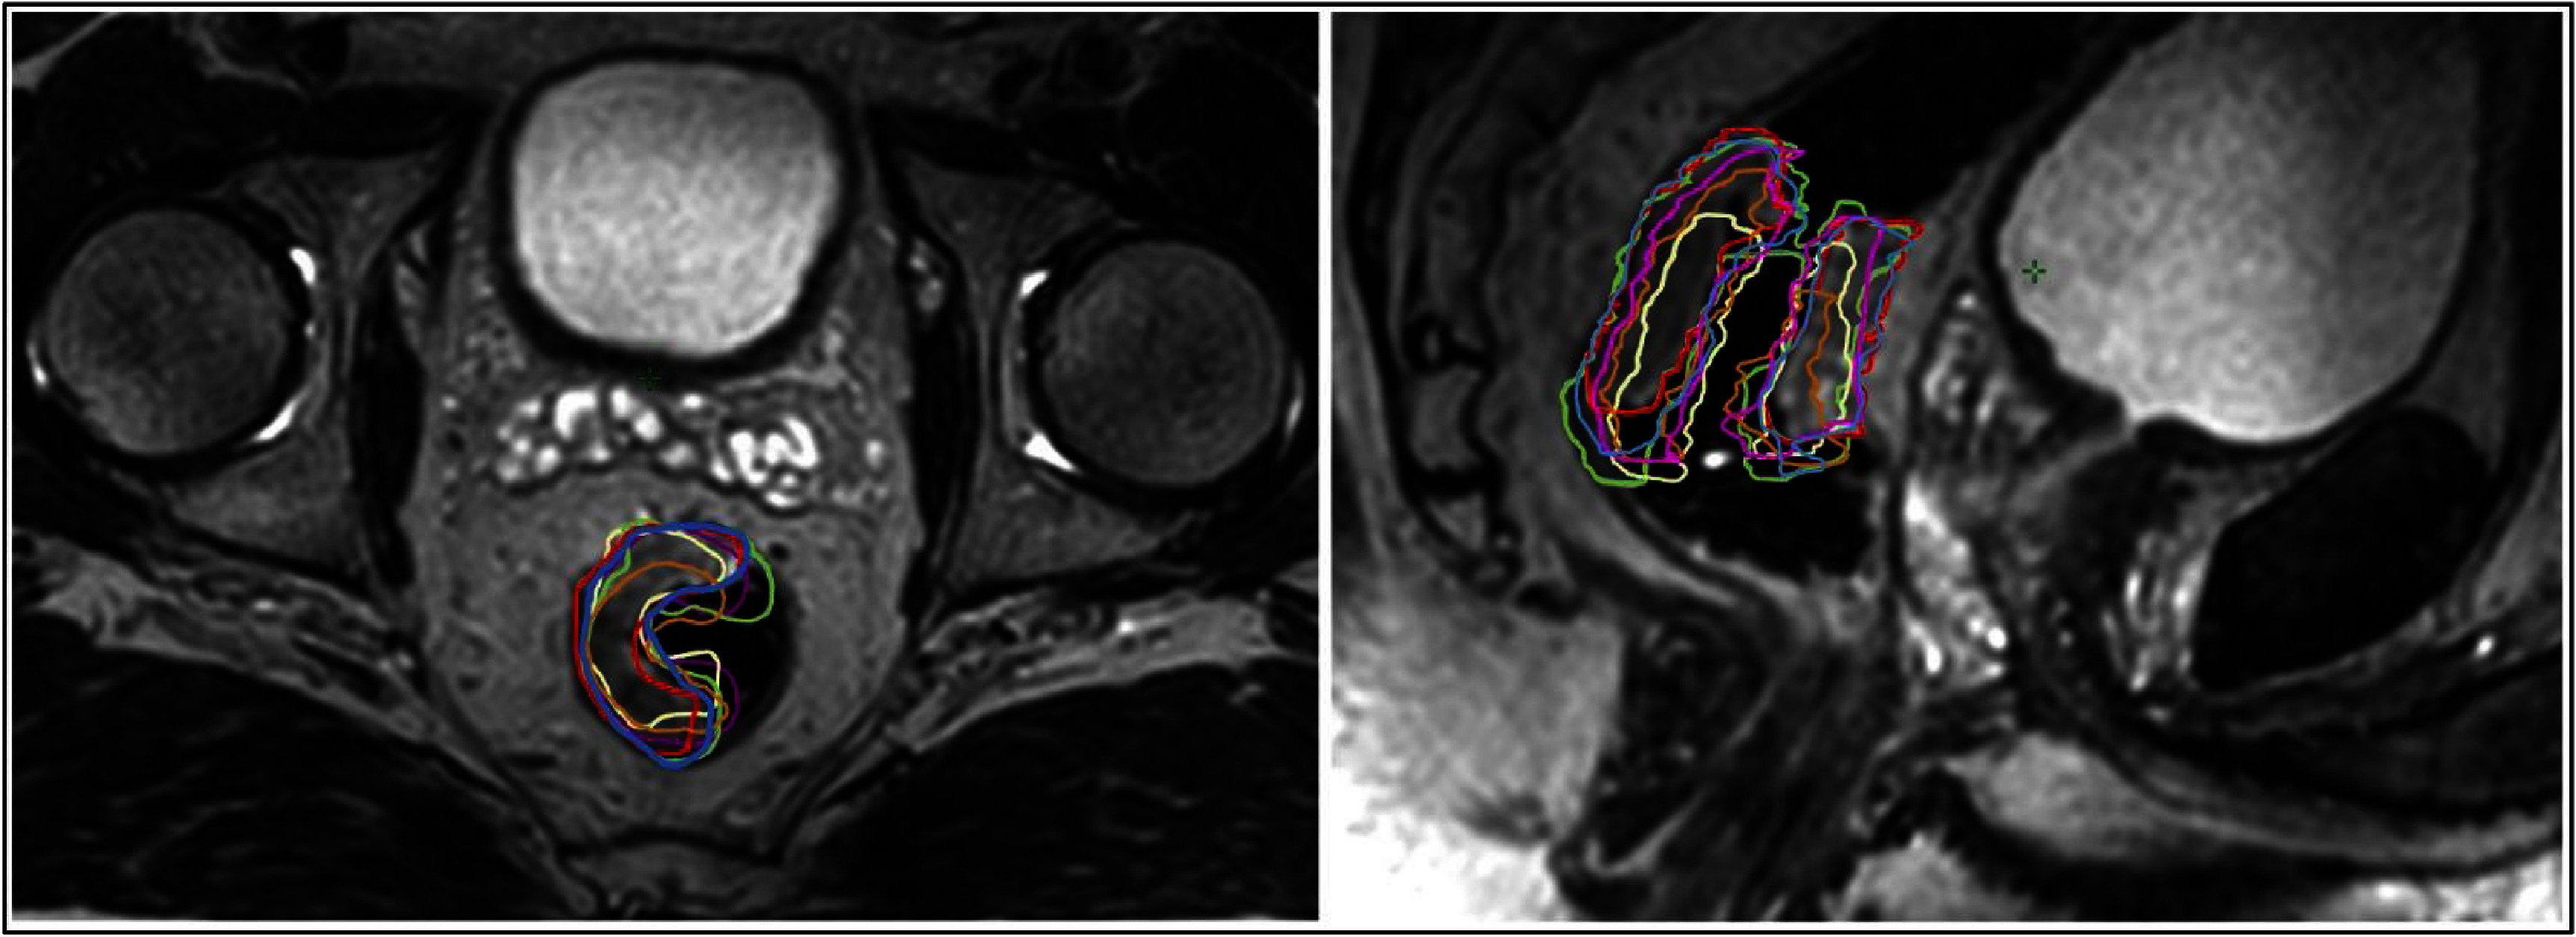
Left: axial view of six GTVp delineations transferred by rigid bone registration to the baseline 3D MRI scan. Right: Same delineations in the sagittal plane. Each color corresponds to a delineation on one of the six MRI scans.
